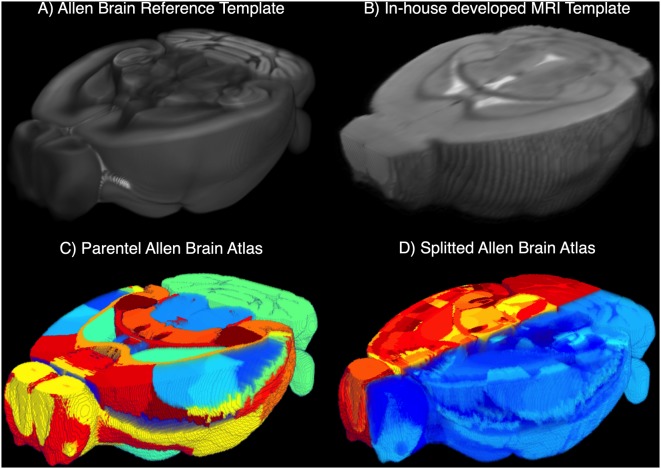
3D cut-outs of the (A) Allen Brain Reference Atlas (ARA) and (B) the in-house developed MRI template (MTPL). The annotations of the ARA *I*_A_(*x*) and the related ARA template (A) are overlaid with the MTPL *I*_T_(*x*) consisting of *N* = 30 T2w. Parental ARA labels *I*_A↑_(*x*) (C) and detailed ARA labels with hemisphere split (D).
